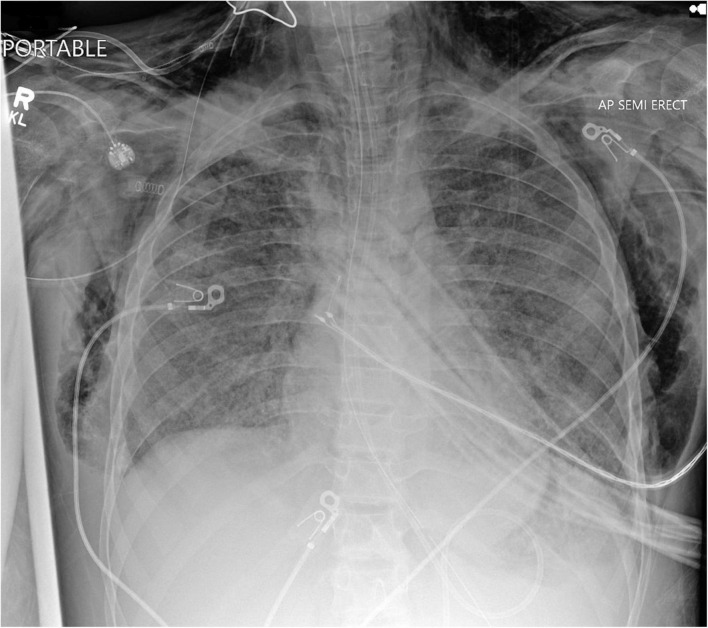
Chest radiograph of a mechanically ventilated pediatric patient, demonstrating subcutaneous emphysema und pneumomediastinum, in the absence of hemodynamic compromise.
